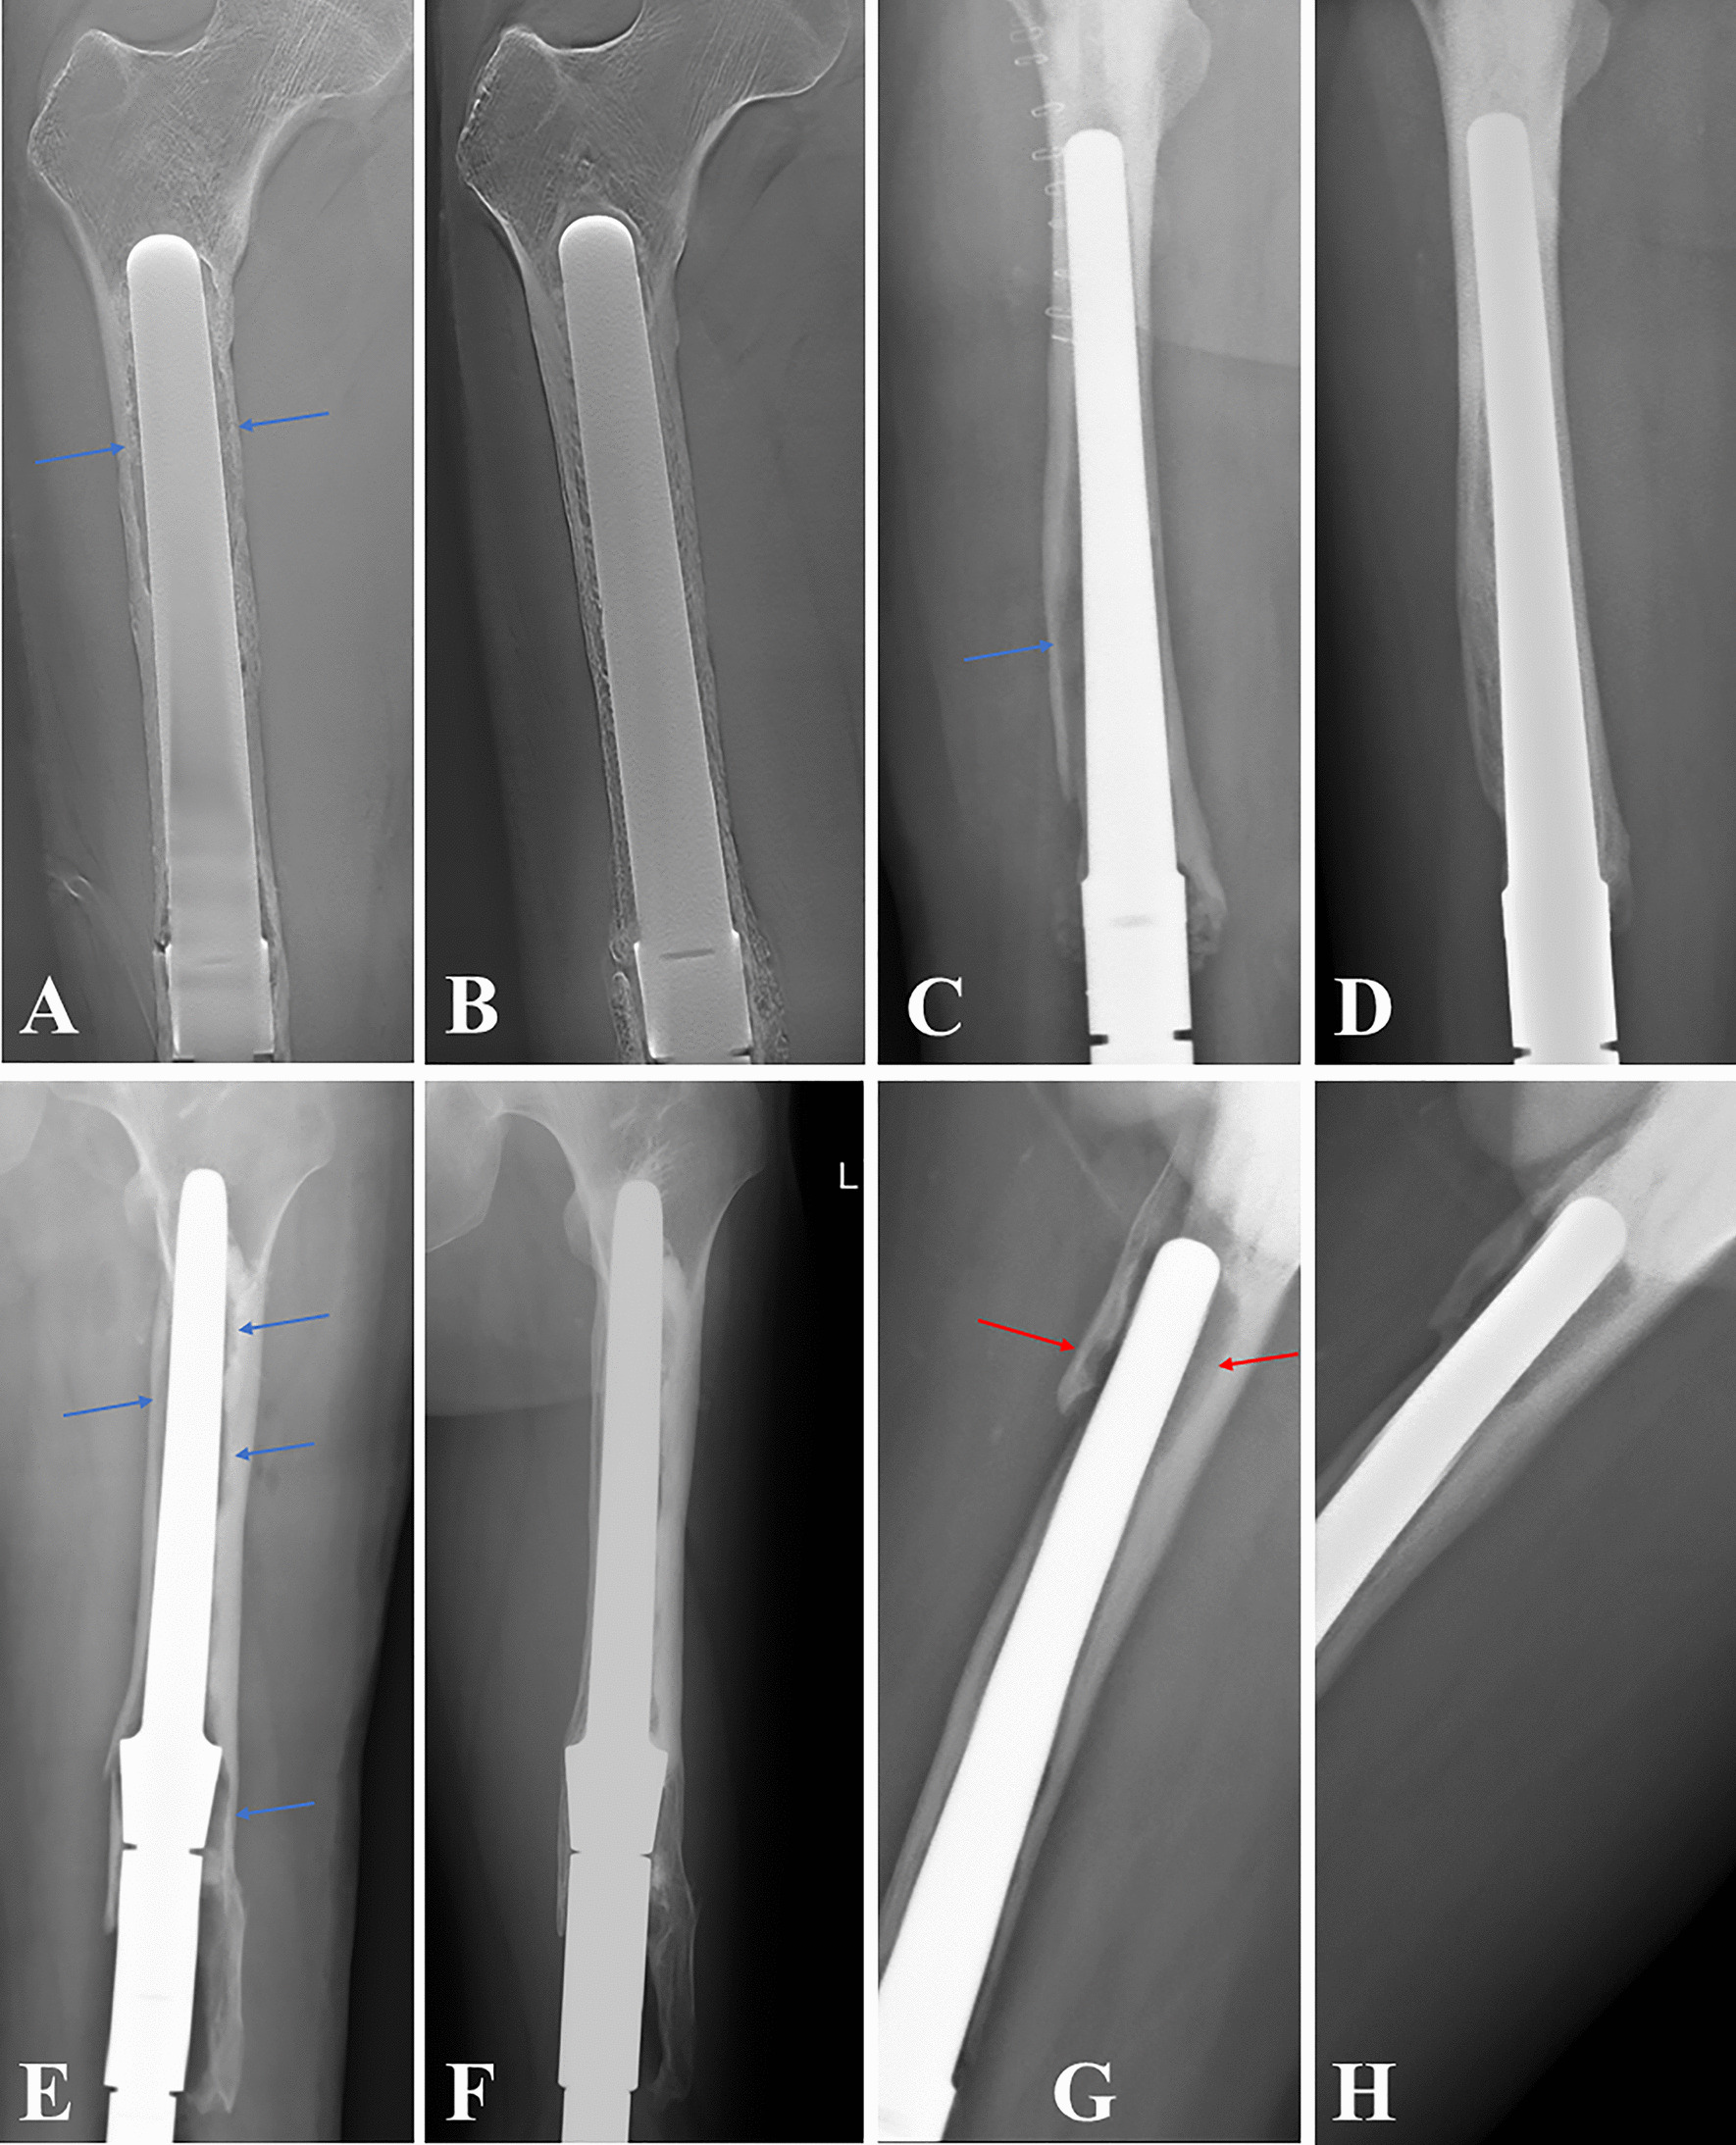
Radiographs demonstrating that the peri-stem remaining defect can be filled or partially restored during the follow-up. The bone defect was filled in the proximal femoral (A, B), distal femur (C, D), and both proximal and distal femur (E, F). The bone defect partially restored (G, H)
